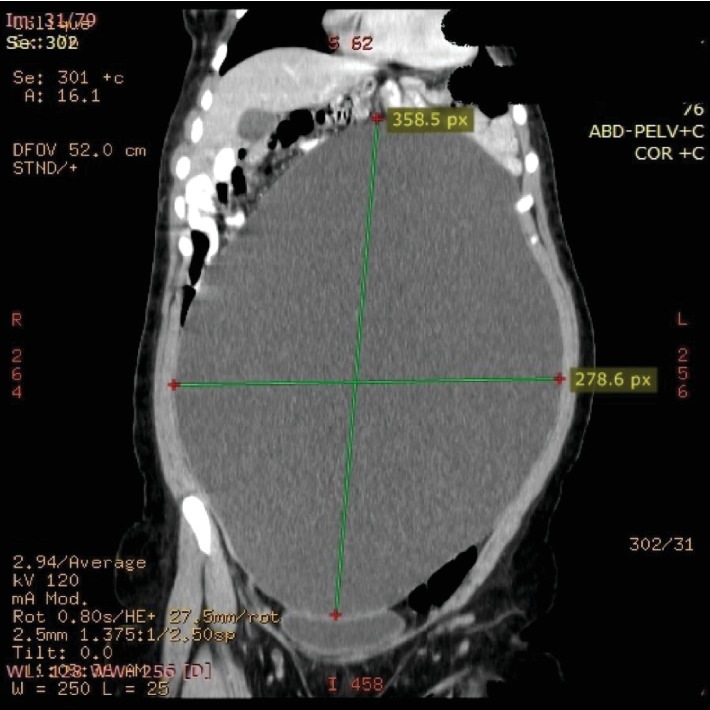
CT scan demonstrating the largest dimensions of the cyst (36 × 28 cm) prior to excision.
